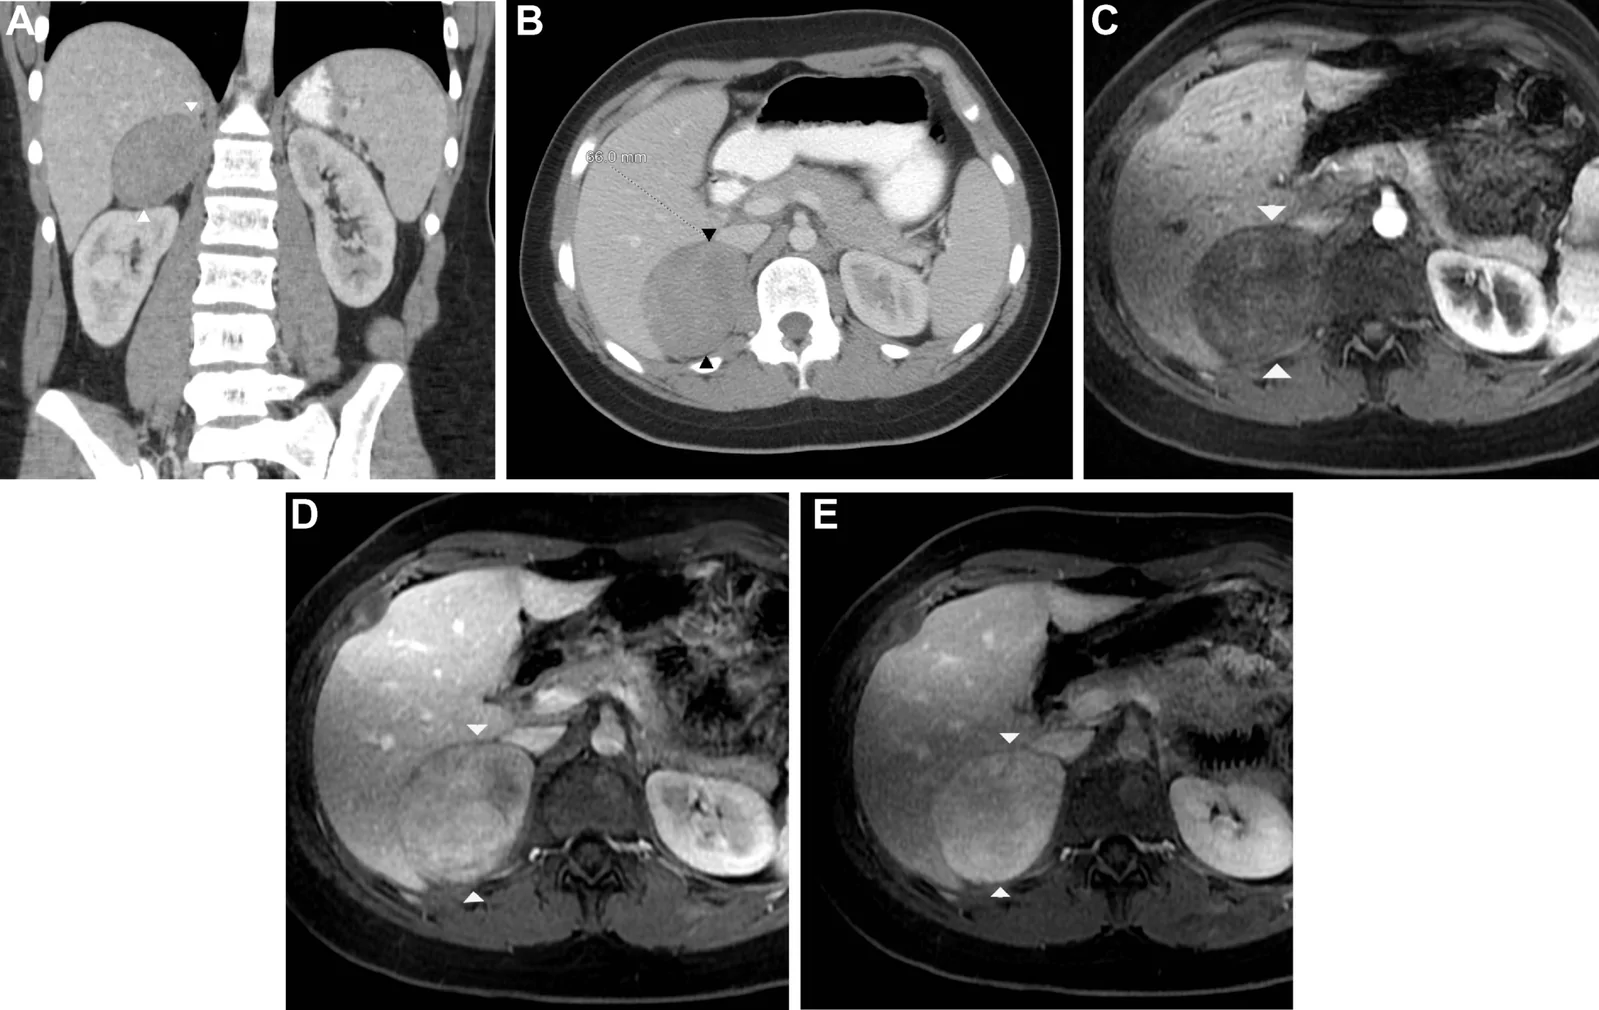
Ganglioneuroma identified incidentally in a 13-year-old female presenting with abdominal pain. a, b Contrast-enhanced CT images of the abdomen reconstructed in (a) coronal and (b) axial planes show a right suprarenal well-defined mass isodense to muscle (*arrowheads*), maximally measuring 6 cm. c-e Post-contrast T1-weighted fat-suppressed MRI in (c) arterial phase, (d) portal venous phase, and (e) 5-min delayed phase demonstrate progressive enhancement of the lesion over time (*arrowheads *indicate mass margins)
